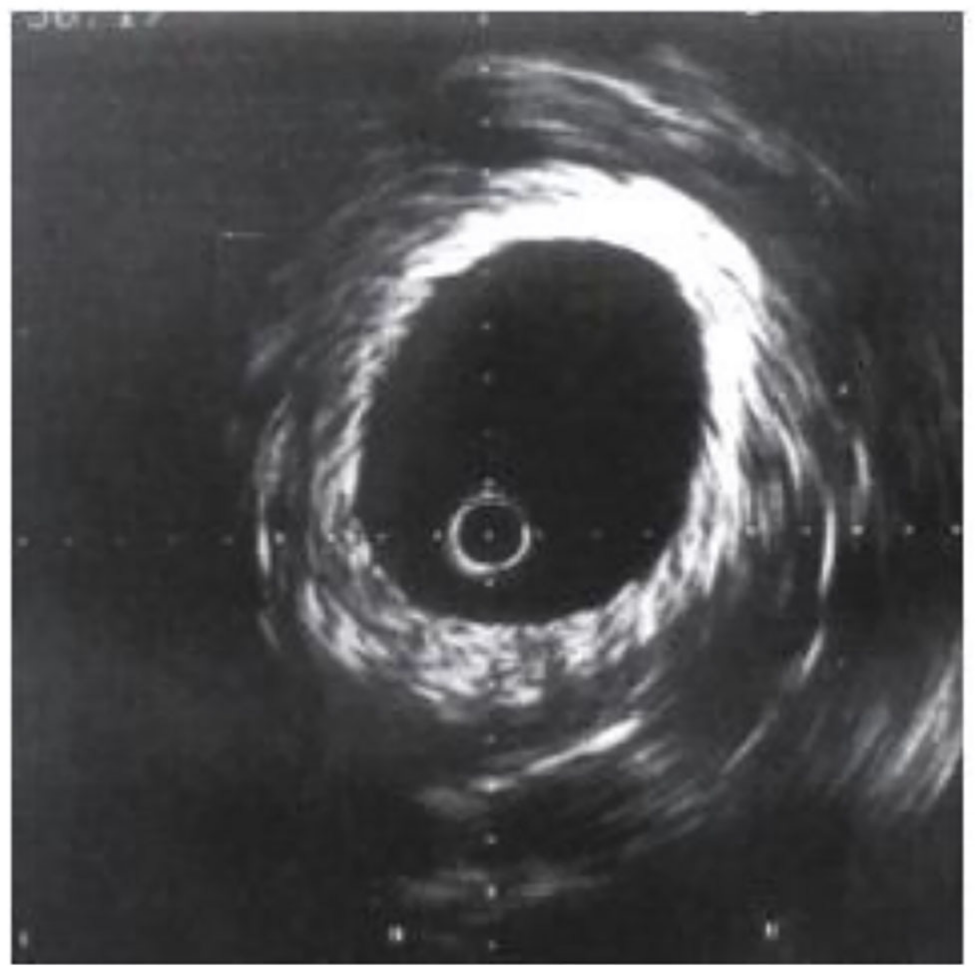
Ultrasound image of non-central position.
